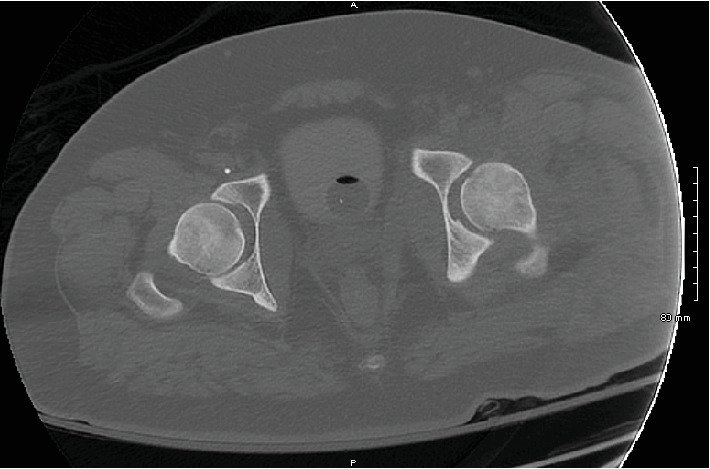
Postreduction axial slice of CT pelvis without contrast demonstrating concentric reduction of the left femoral head.
